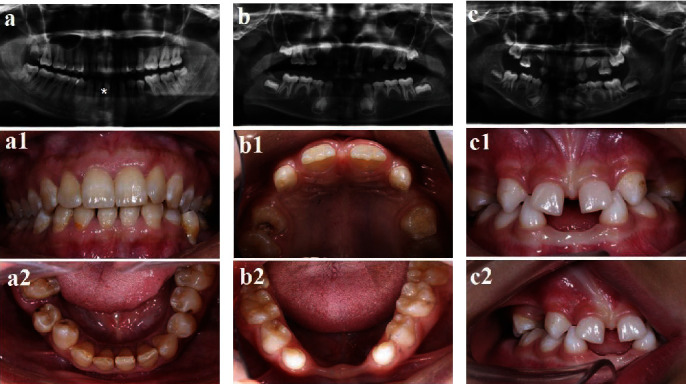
Dental phenotype and panoramic radiographs of the patients. (a–c) Panoramic radiographs of II:2, III:1, and III:2. (a1, a2; b1, b2; c1, c2) Intraoral photos of II:2, III:1, and III:2.
